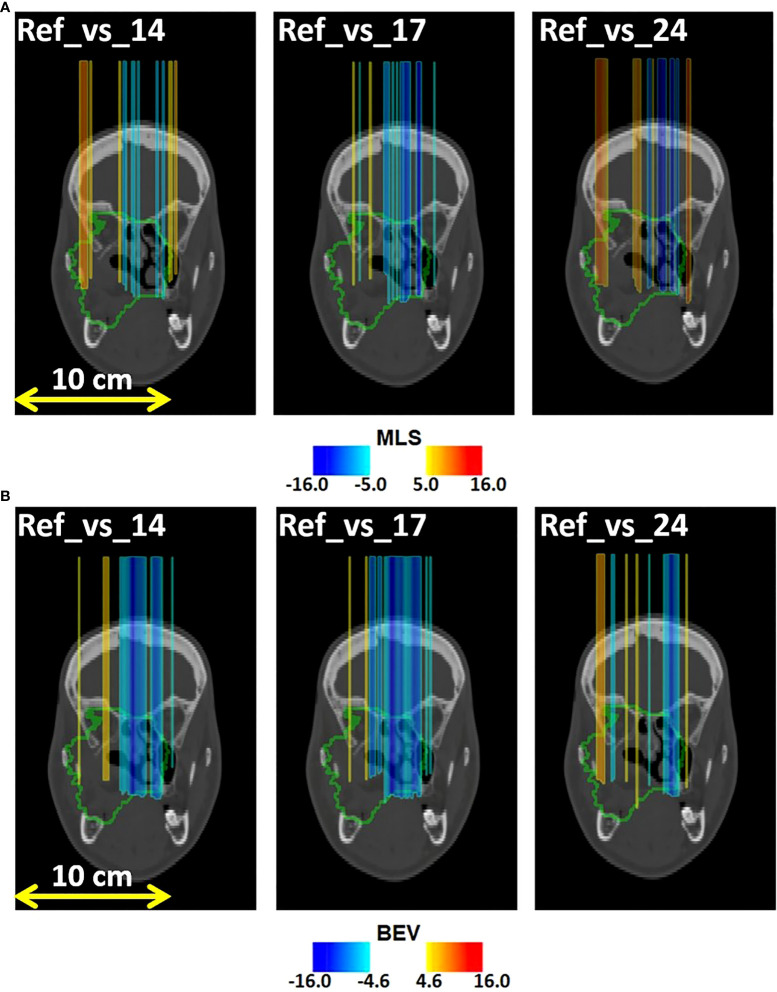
Coronal views of the control CT of patient 007P, with superimposed the (A) MLS outliers map *O_MLS_
